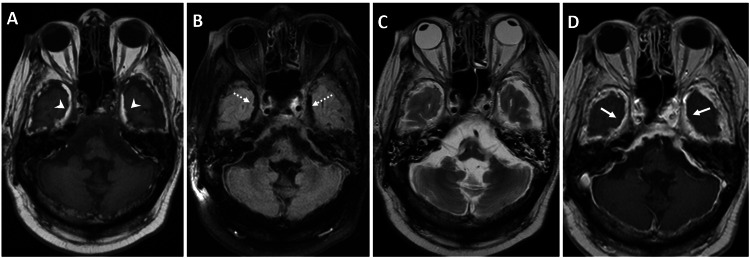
MRI characterization of dural fat deposition with corresponding signal changes and diffuse pachymeningeal enhancement. An MRI of the brain was performed in a 66-year-old male with a medical history of altered mental status, in the context of known severe mental retardation, cerebral palsy, and a VP shunt. (A) Axial T1-weighted unenhanced MRI brain images show dural T1 hyperintensity along the middle cranial fossa (white arrowheads), correlating with the fat density seen on the CT scan. (B) Axial FLAIR image shows a drop in signal corresponding to the dural T1 hyperintensity (dashed white arrows). (C) Axial T2-weighted MRI images show barely appreciable dural T2 hyperintensity along the middle cranial fossa, separable from the T2 hyperintense CSF signal. (D) Axial T1-weighted contrast-enhanced MRI brain image, after intravenous injection of 20 mL of gadoteridol (ProHance; Bracco, Milan, Italy), shows diffuse pachymeningeal dural enhancement (solid white arrows). MRI, magnetic resonance imaging; CT, computed tomography; FLAIR, fluid-attenuated inversion recovery; CSF, cerebrospinal fluid; VP, ventriculoperitoneal
